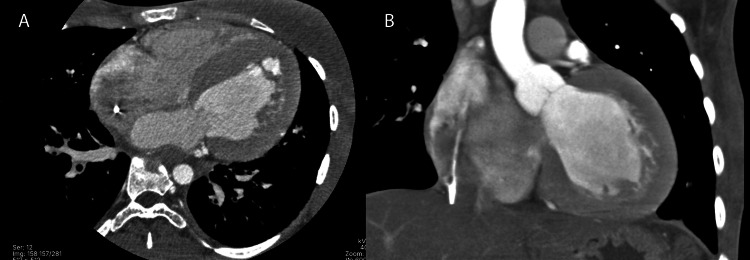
Contrast-enhanced thoracic CT angiography A) Sagittal view shows concentric left ventricular hypertrophy with septal predominance. B) Axial view reveals moderate dilation of the right atrium and the pulmonary artery trunk. Additionally, a hyperdense intracavitary mass is identified, located in the right atrium and adjacent to the endocardial wall.
